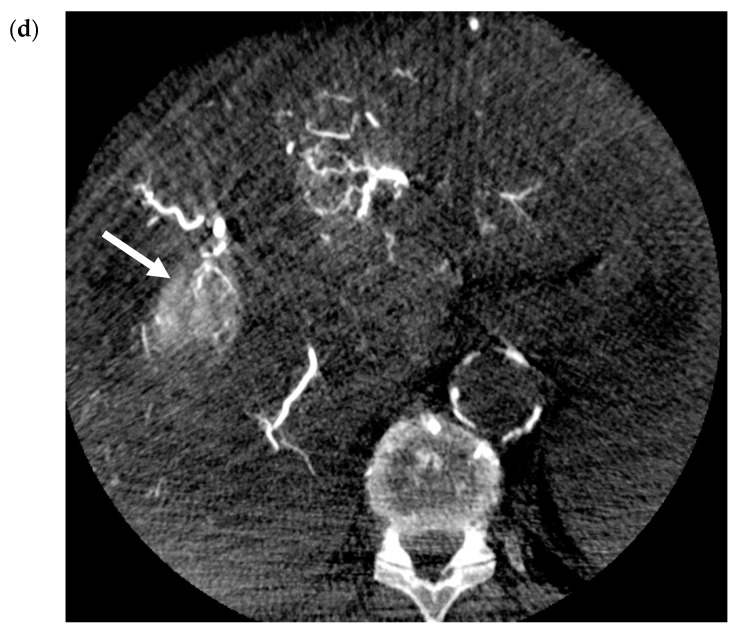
Transarterial Embolization (TAE) of HCC. (a) Pre-procedure triphasic contrast-enhanced CT in the arterial phase. Mass with arterial hyperenhancement in segment 8 of the right lobe of liver (white arrow), consistent with hepatocellular carcinoma (HCC). (b) Digital subtraction angiogram of the right hepatic artery pre-bland embolization. Mass (white arrow) is supplied by the segment 8 branch of the right hepatic artery (black arrow). (c) Digital subtraction angiogram of the right hepatic artery post-bland embolization of the segment 8 branch of the right hepatic artery. A combination of 40-120-µm microspheres and 100-µm polyvinyl alcohol (PVA) particles were used. Angiogram demonstrates the stasis of iodinated contrast in the segment 8 arterial branch with pruning of the distal branches and no opacification of the mass. (d) Intra-procedural cone-beam CT immediately post-bland embolization. Retention of iodinated contrast and particles in the treated tumor (white arrow) identical to pre-procedure post-contrast images indicative of complete tumor coverage.
